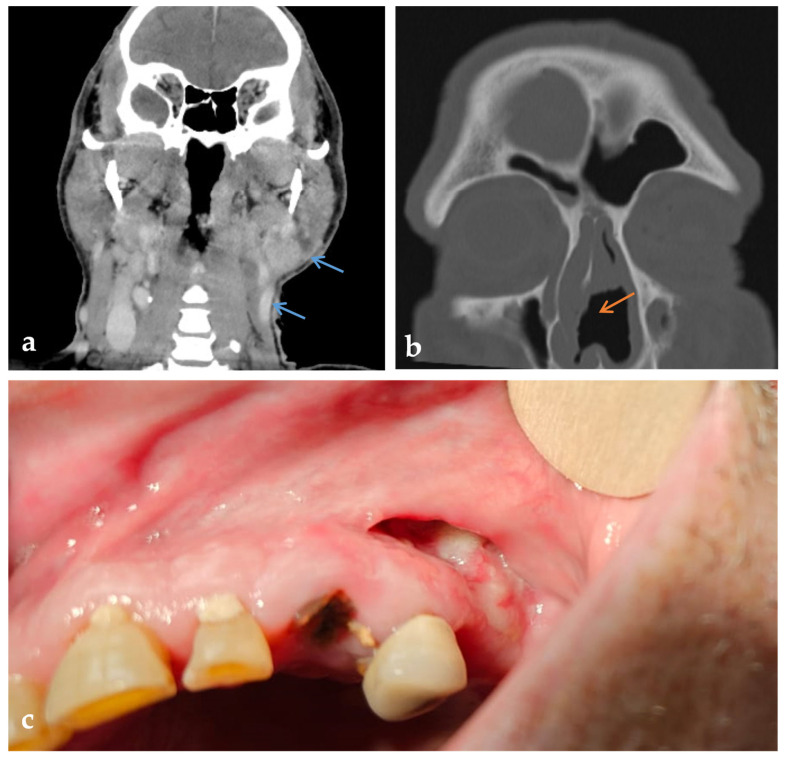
Radiologic and intraoral findings in PR3-ANCA-positive cocaine-induced vasculitis (CIV). (a) Coronal contrast-enhanced CT showing soft tissue lesions with increased enhancement in the left supraclavicular and parotid region (blue arrows). (b) Axial CT of the paranasal sinuses with perforation of the nasal septum (orange arrow). (c) Intraoral view showing mucosal ulceration and exposed bone in the upper left oral vestibule.
